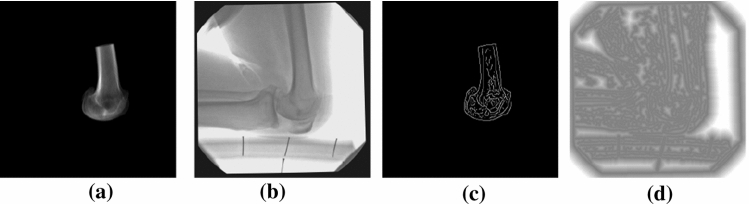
a, b show an example of 2D projection of a CT image and a fluoroscopy image to be registered. c Represents the binary edge image of the 2D projection of the CT image. The distance to the edge position of the fluoroscopy image is shown in d
